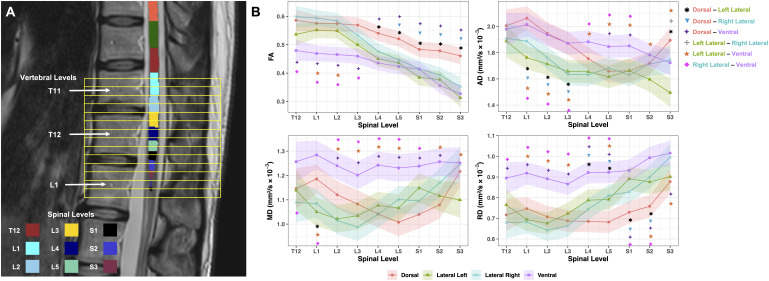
(A) Representative T2-weighted sagittal image of one healthy participant (33-year-old female), with axial diffusion field-of-view (FOV) overlaid in yellow with registered spinal levels. The lumbosacral (neurological) spinal levels typically correspond spatially with vertebral levels ~T11–L1, though there is some inter-subject variability that is accounted for when registering template spinal levels to individual subject images. (B) Marginal mean by white matter column (dorsal, left lateral, right lateral, and ventral) in DTI measures fractional anisotropy (FA), axial diffusivity (AD), radial diffusivity (RD), and mean diffusivity (MD) across all spinal levels in healthy controls. Shapes (✱▼✦✢★◆) indicate an FDR-adjusted p-value (correction with Benjamini–Hochberg method across spinal levels) < 0.05 for the marginal mean difference between the different white matter columns for a given spinal level.
